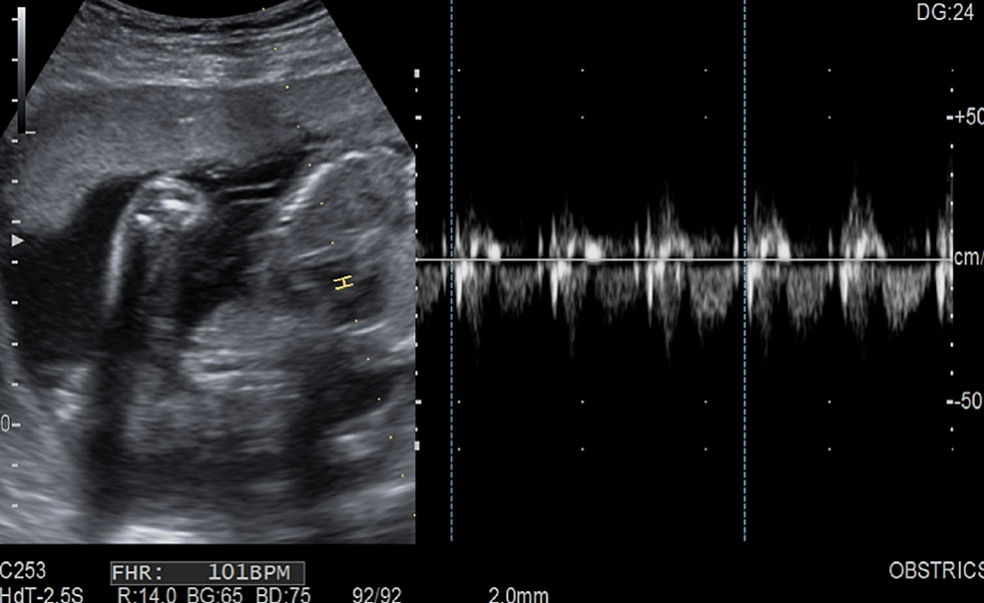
Transabdominal ultrasound shows an intrauterine live fetus with a fetal heart rate of 101 bpm. bpm: beats per minute.
